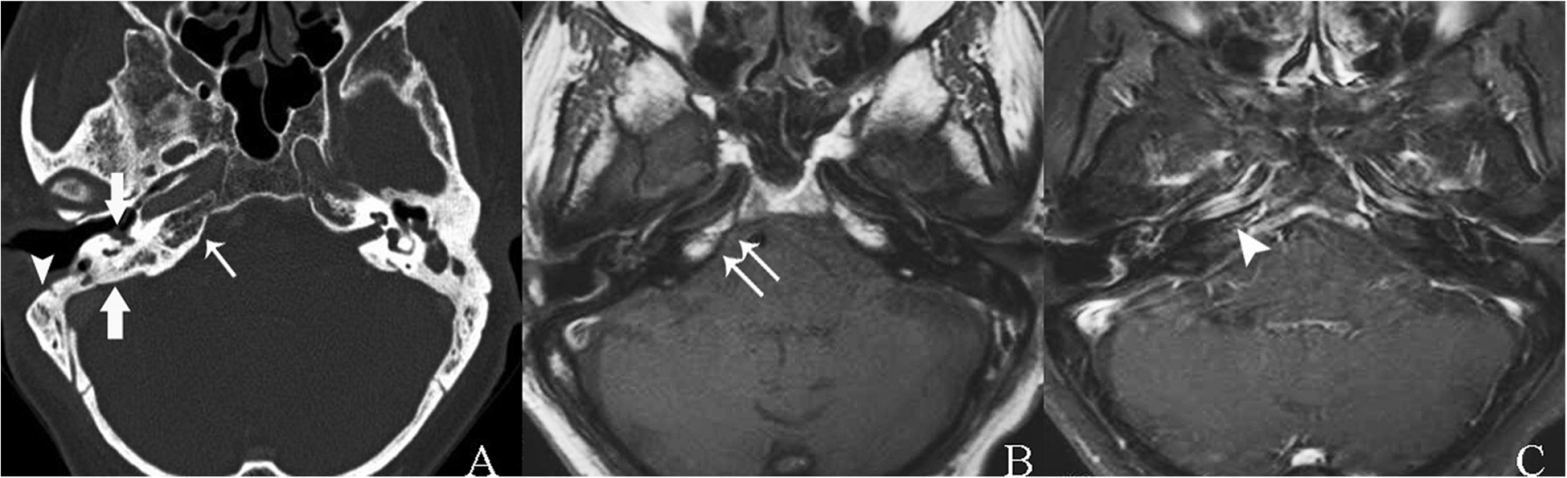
Axial computed tomography and magnetic resonance imaging of temporal bone. A. Thin-slice bone algorithm non-contrast computed tomography revealed partial opacified right middle ear cavity and residual mastoid air cells (thick arrows) as a result of chronic otomastoiditis, and post-mastoidectomy status seen as localized defect along the anterolateral wall of right mastoid air cells (arrowhead). Poor pneumatization of right petrous apex (arrow) was noted and was later confirmed by magnetic resonance imaging. B. Spin echo T1-weighted magnetic resonance imaging demonstrated high signal intensity of fatty marrow (arrows), as a normal variant. C. Postgadolinium-pentetic acid spin echo T1-weighted with fat suppression magnetic resonance imaging reveals subtle evidence of right petrous apicitis seen as slightly asymmetrical prominent enhancement as compared to the normal left side (arrowhead).
